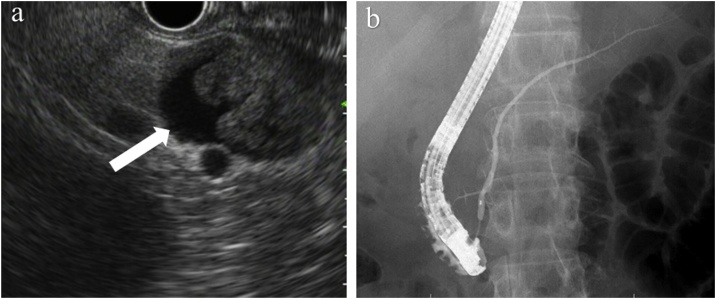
Endoscopic imaging: A. Endoscopic ultrasonography (EUS) showed a cyst with multiple high-echoic lesions in the pancreatic tail (arrow). B. Endoscopic retrograde cholangiopancreatographic image showed a normal main pancreatic duct and no communication between the main pancreatic duct and the cystic lesion.
